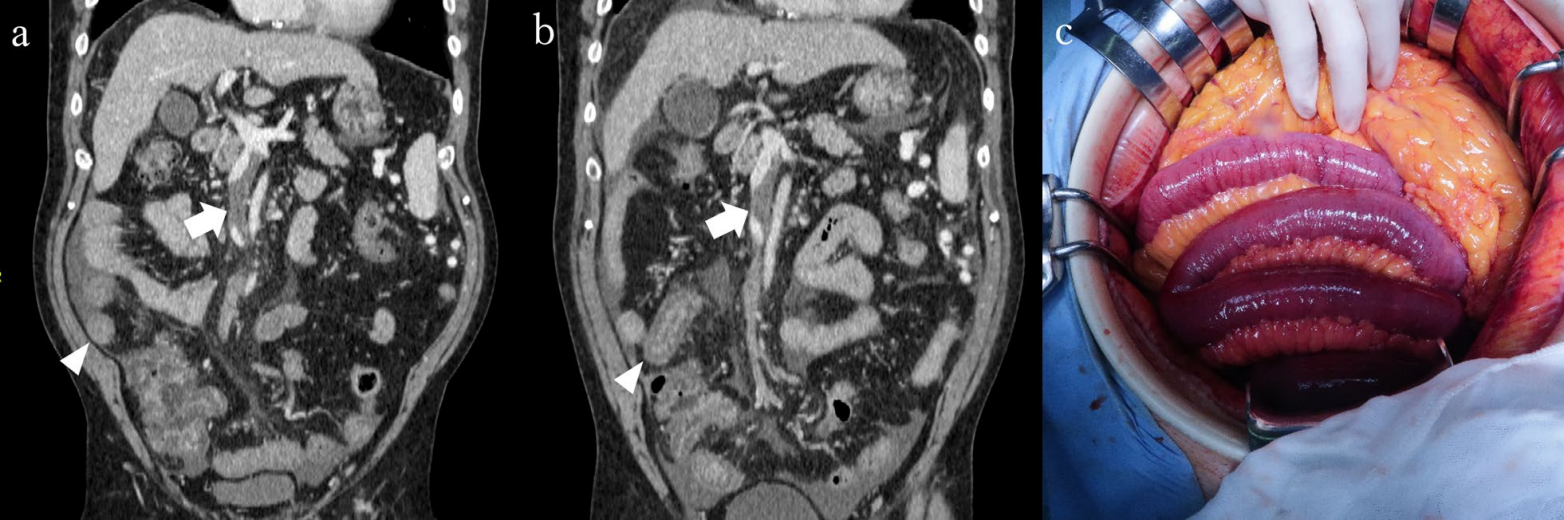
a CE-CT findings on admission (coronal view) of Case 4. Thrombus occludes the SMV but not the splenic vein (arrow). Ileum edema at short range and moderate ascites are observed (arrowhead). b CE-CT findings at the time of worsening symptoms (coronal view) of Case 4. The thrombus in SMV has not changed (arrow). Ileum edema is worsening (arrowhead). c Intraoperative findings. A gangrenous small bowel measuring 50 cm is resected
